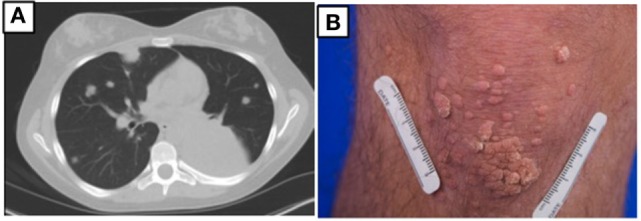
(A) Chest CT prior to hematopoietic stem cell transplant for patient 1 showing multiple masses due to EBV lymphoproliferative disease. (B) Multiple warts on the knee for patient 2.
